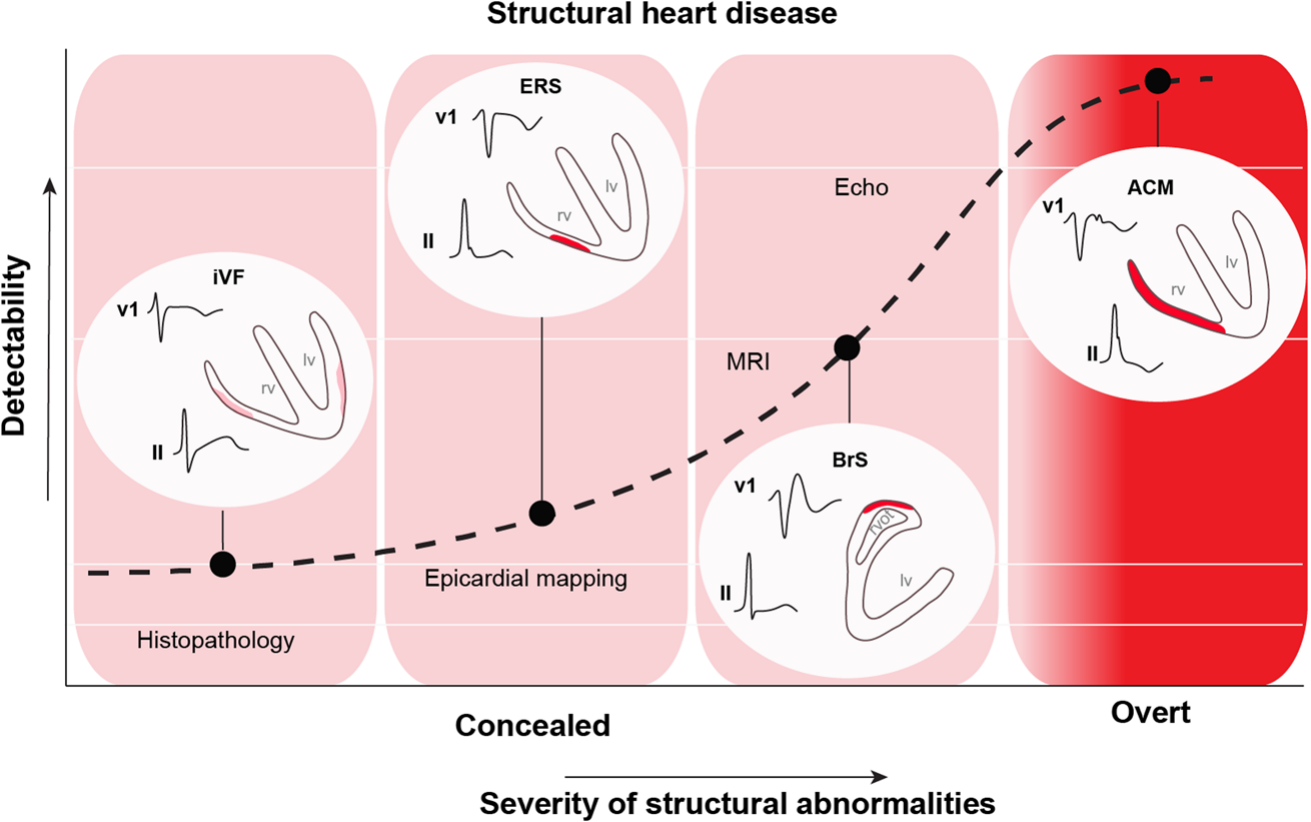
Detectability and severity of structural abnormalities in BrS, ERS, and ACM with respect to cardiac diagnostic modalities. ACM indicates arrhythmogenic cardiomyopathy; BrS, Brugada syndrome; ERS, early repolarization syndrome; iVF, idiopathic ventricular fibrillation; and MRI, magnetic resonance imaging. Adapted from Boukens et al^94^ with permission. Copyright © 2020 Elsevier.
